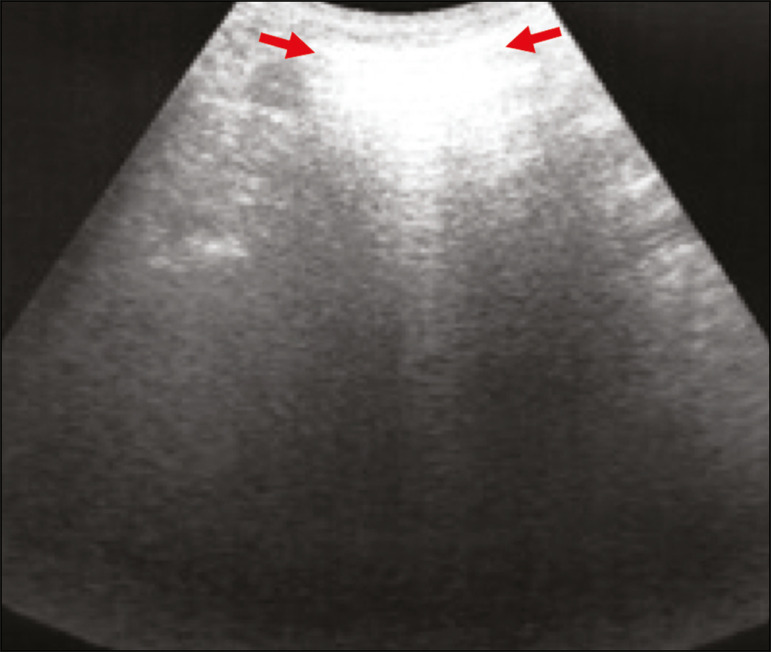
B-mode chest ultrasound demonstrates subcutaneous emphysema (arrows).
